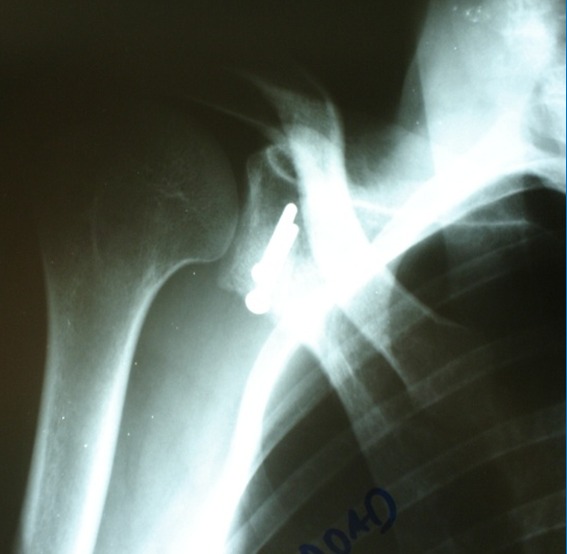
Radiographie de l’épaule de face objectivant la position de la butée fixée par deux vis
